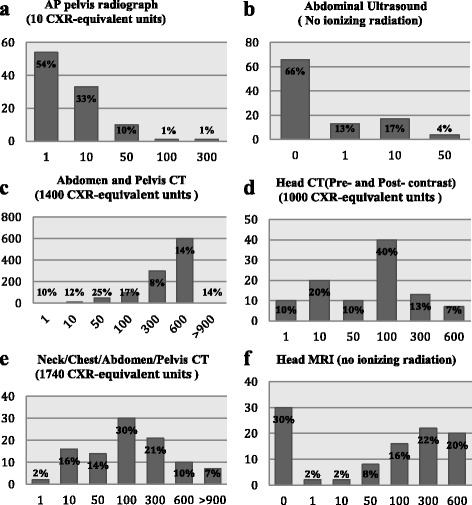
Estimation of the effective dose of a 5-year-old child during a PA chest x-ray (equivalent units). a) AP pelvic radiograph (10 CXR-equivalent units); b) abdominal ultrasound (No ionizing radiation); c) abdomen and pelvic CT (1300 CXR-equivalent units); d) head CT (Pre- and Post- contrast) (1100 CXR-equivalent units); e) neck/chest/abdomen/pelvic CT (1700 CXR-equivalent units); f) head MRI (no ionizing radiation)
